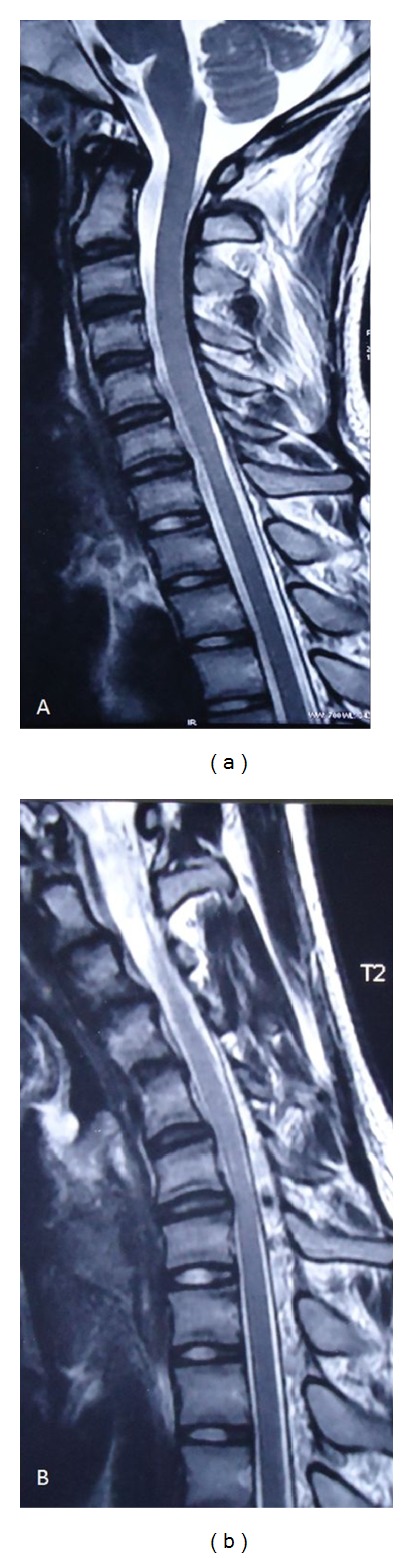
(a) Neutral position MRI of cervical spine T2W image reveals mild focal atrophy of cervical cord at C6-C7 levels with loss of attachment between the posterior dural sac and subjacent lamina. (b) Flexion MRI of cervical spine T2W image reveals characteristic anterior displacement of the posterior wall of cervical dural canal reaching up to spinal cord. Prominent posterior epidural spaces with multiple flow voids suggestive of prominent venous plexus are present.
